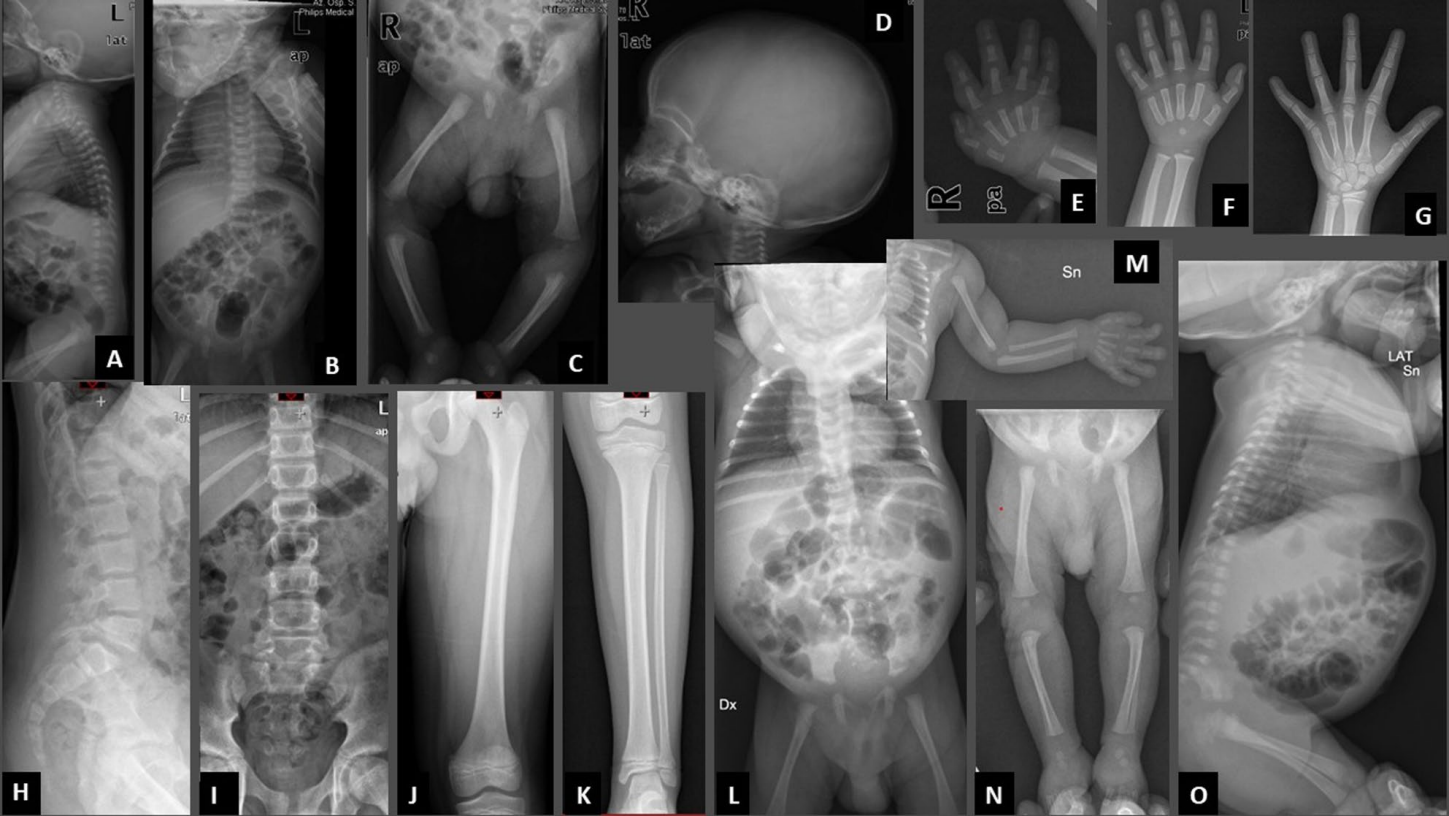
Radiographs of patients 1 and 2. X-rays of patient 1 at birth: slender and elongated long bones, with diaphyseal constriction and cortical thickening (C); tall vertebrae, with reduced anteroposterior diameter (A, B); thin metacarpals and phalanges (E). Left wrist and hand X-ray of patient 1 at the age of 2 years: Skeletal maturation assessment on X-rays of the left wrist and hand with the Greulich and Pyle method: marked delay, with bone age of 9 months at the chronological age of 2 years (F). X-rays of patient 1 at the age of 12 years: delayed skeletal maturation, with a bone age of 9–10 years. Slightly tall lumbar vertebral bodies, thin long bones (G-K). X-rays of patient 2 at birth: slightly slender bones and lightly flared metaphyses of the distal femur and proximal tibia without other evident signs of skeletal dysplasias (L-O)
